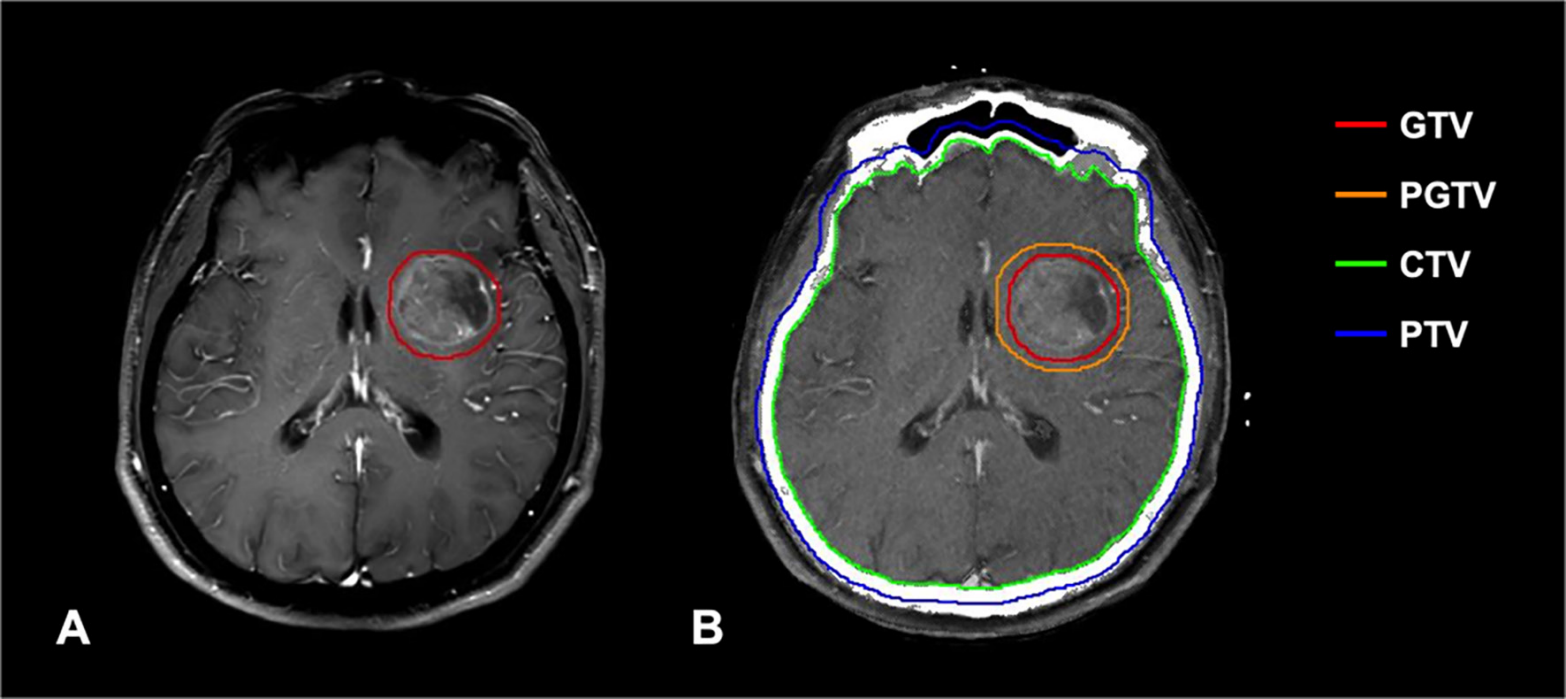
(A) T1-weighted contrast-enhanced MRI shows high signal intensity in the metastatic lesion. (B) Delineation of important RT volumes (GTV, PGTV, CTV, and PTV) was based on contrast-enhanced MRI.
